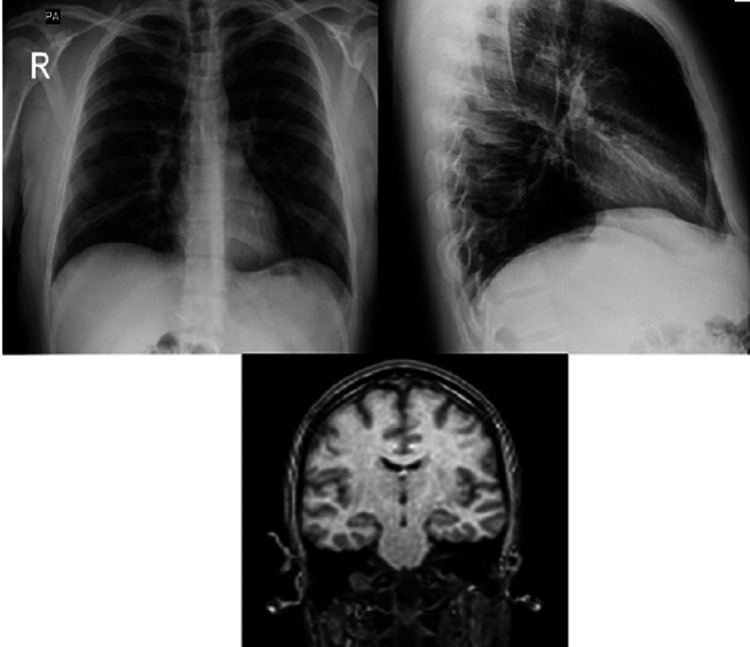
Anteroposterior and lateral views of chest X-ray and an MRI of the brain.
